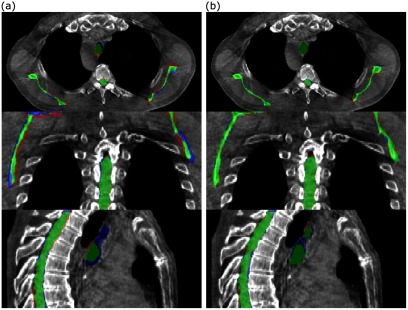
Comparison of (a) rigidly aligned and (b) deformed structures with the directly outlined CBCT gold standard for a single patient. Green represents areas covered by both sets of structures, blue represents areas covered by the directly outlined structures only and red represents areas covered by the rigidly aligned/deformed structures only.
